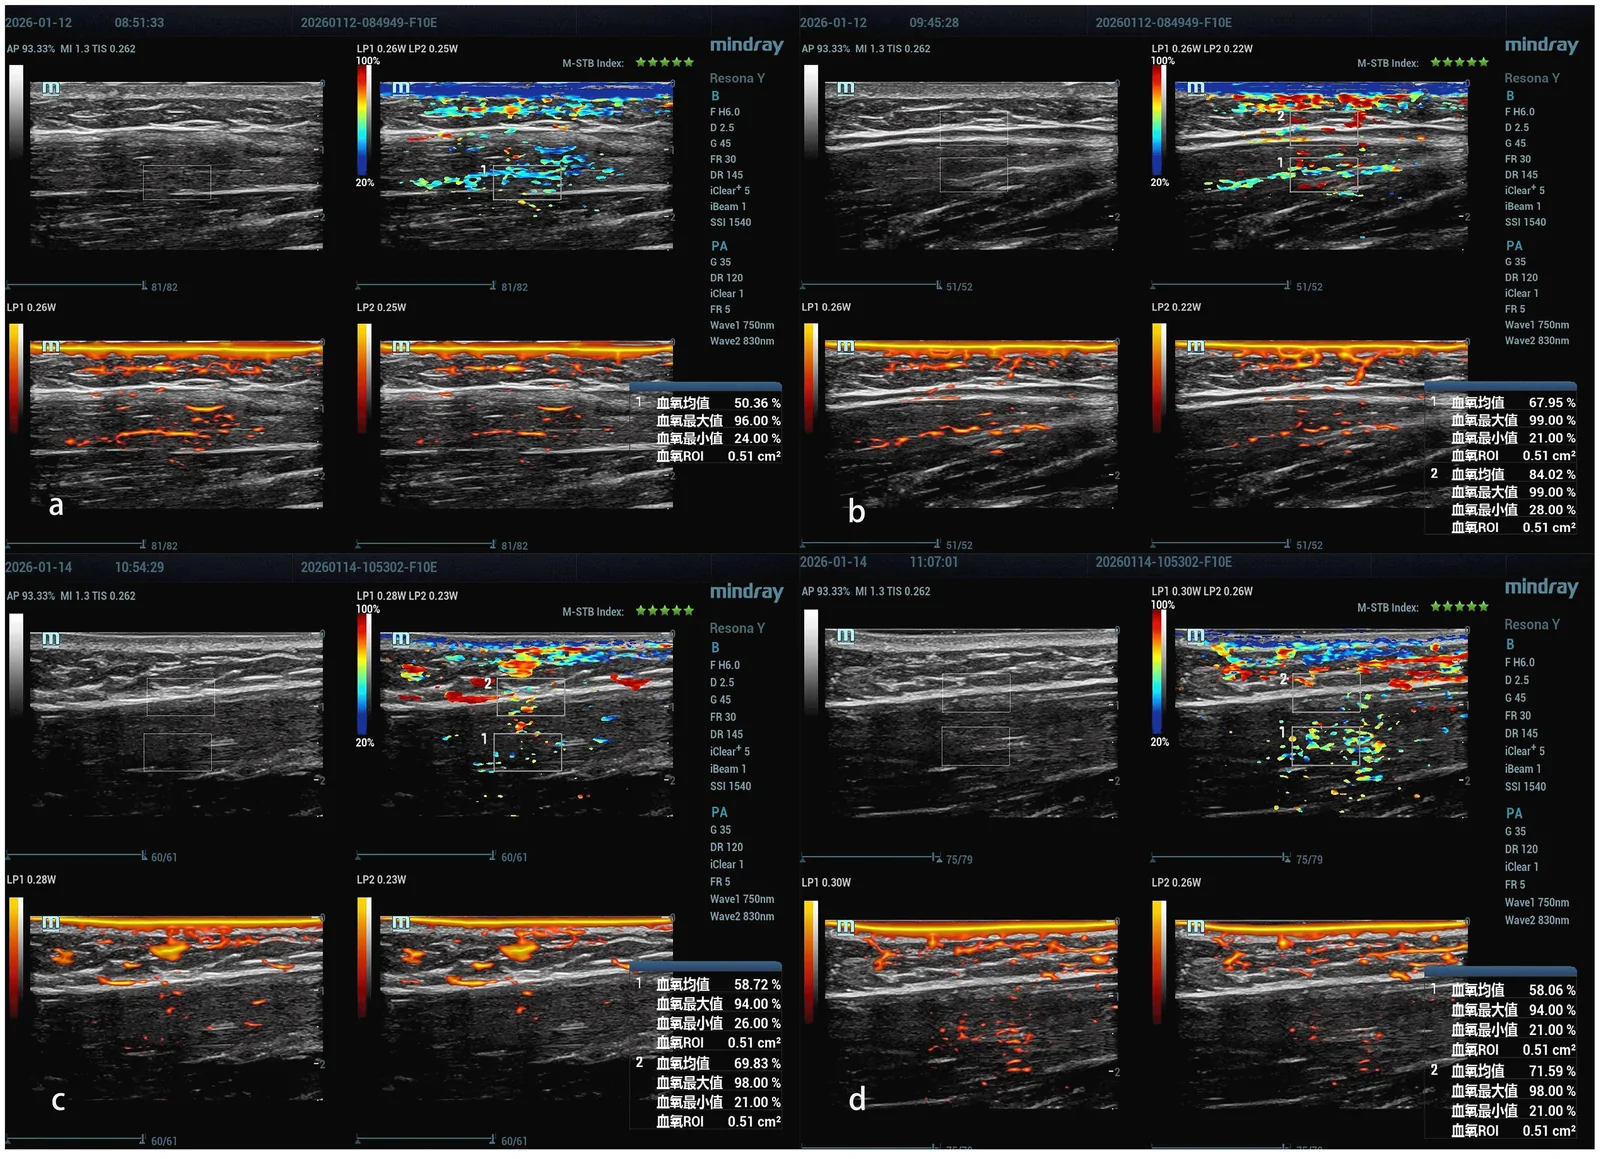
Upper left: Grayscale B-mode anatomical ultrasound for muscle layer identification (brachioradialis & extensor carpi radialis longus annotated). Upper right: Coregistered PAI pseudocolor SO_2_ functional heatmap (jet colormap: red = high oxygen saturation, blue = low saturation). Lower left: Raw grayscale photoacoustic signal intensity captured at 750 nm excitation wavelength for signal quality assessment. Lower right: Raw grayscale photoacoustic signal intensity captured at 830 nm excitation wavelength for signal quality assessment. (a) A vertical section of Shousanli (LI10) from a 21−year−old male volunteer. SO_2_ detected by PAI was 50.36%. (b) A vertical section of Shousanli (LI10) from a 21−year−old male volunteer. Post−acupuncture SO_2_ detected by PAI was 67.95%. (c) A vertical section of Shousanli (LI10) from a 20−year−old female volunteer.SO_2_ detected by PAI was 54.72%. (d) A vertical section of Shousanli (LI10) from a 20−year−old female volunteer. Post−acupuncture SO_2_ detected by PAI was 58.06%.Color scale represents SO_2_ percentage, with red/warm colors indicating higher oxygen saturation and blue/cool colors indicating lower oxygen saturation. Pixels with signal-to-noise ratio < 10 dB were excluded during ROI mean SO_2_ calculation to eliminate imaging noise interference.
